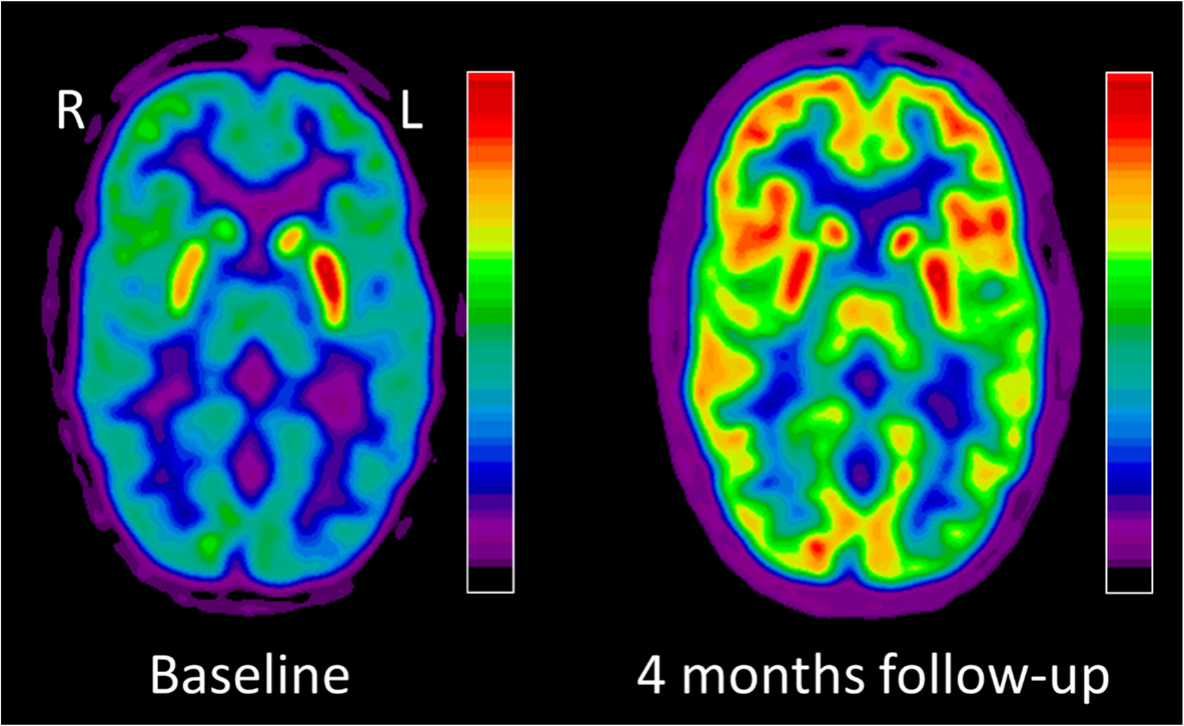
Images of the cerebral FDG positron emission tomography/ computer tomography (PET/CT). At baseline prior to plasmapheresis, the patient demonstrated a moderate to severe hypermetabolism of the left striatum. The cortex revealed hypometabolic signal. Metabolic changes were completely normalized at follow-up four months later
